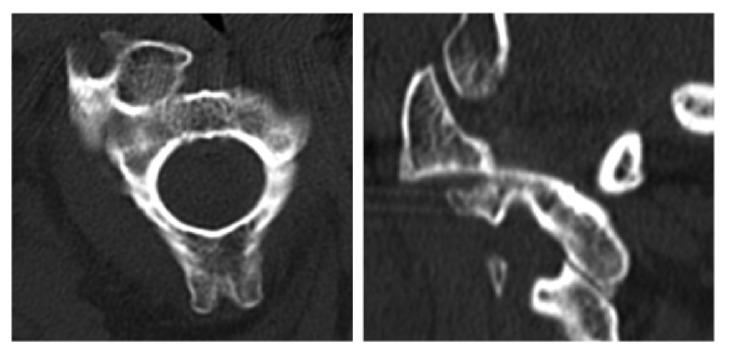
Transversal and sagittal CT imaging of the atlantoaxial facet joints, showing a rotatory injury of the atlantoaxial complex, type II by Fielding [9] demonstrating rotation of C1 around the left atlantoaxial facet joint.
